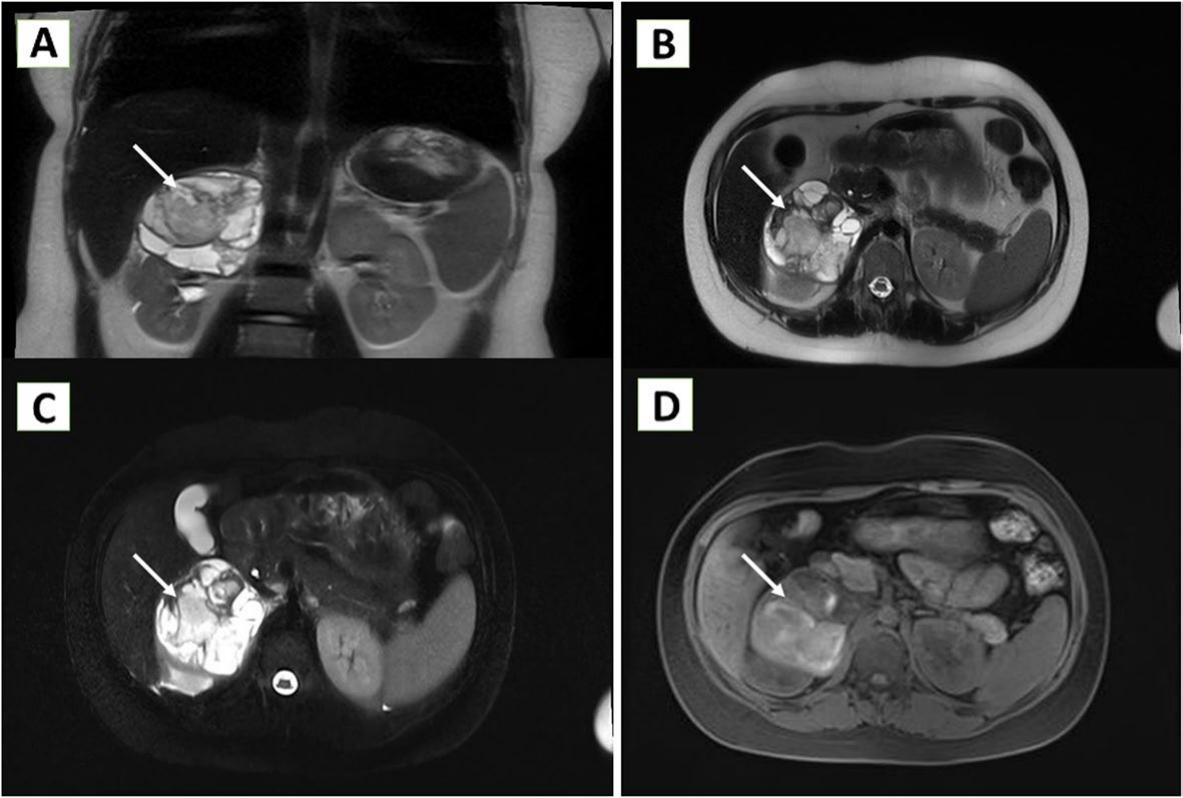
A T2-weighted, coronal view. B T2-weighted, axial view. C Fat suppressed sequence, axial view. D Gadolinium enhanced T1, axial view. The formation shows strongly heterogeneous MR-characteristic, mainly cystic (T2-hyperintense) and with hemorrhagic content (T1-hyperintense); there is presence of a relatively thick capsule (possibly a pseudocapsule due to a highly thin and peripherally dislocated parenchyma) and fine septa. After application of contrast material, there is an amplification of the signal at the periphery (capsule/pseudocapsule) of the lesion and in the septa. Mass effect on the upper pole of the right kidney, right hepatic lobe, inferior vena cava, and right renal vein is present
